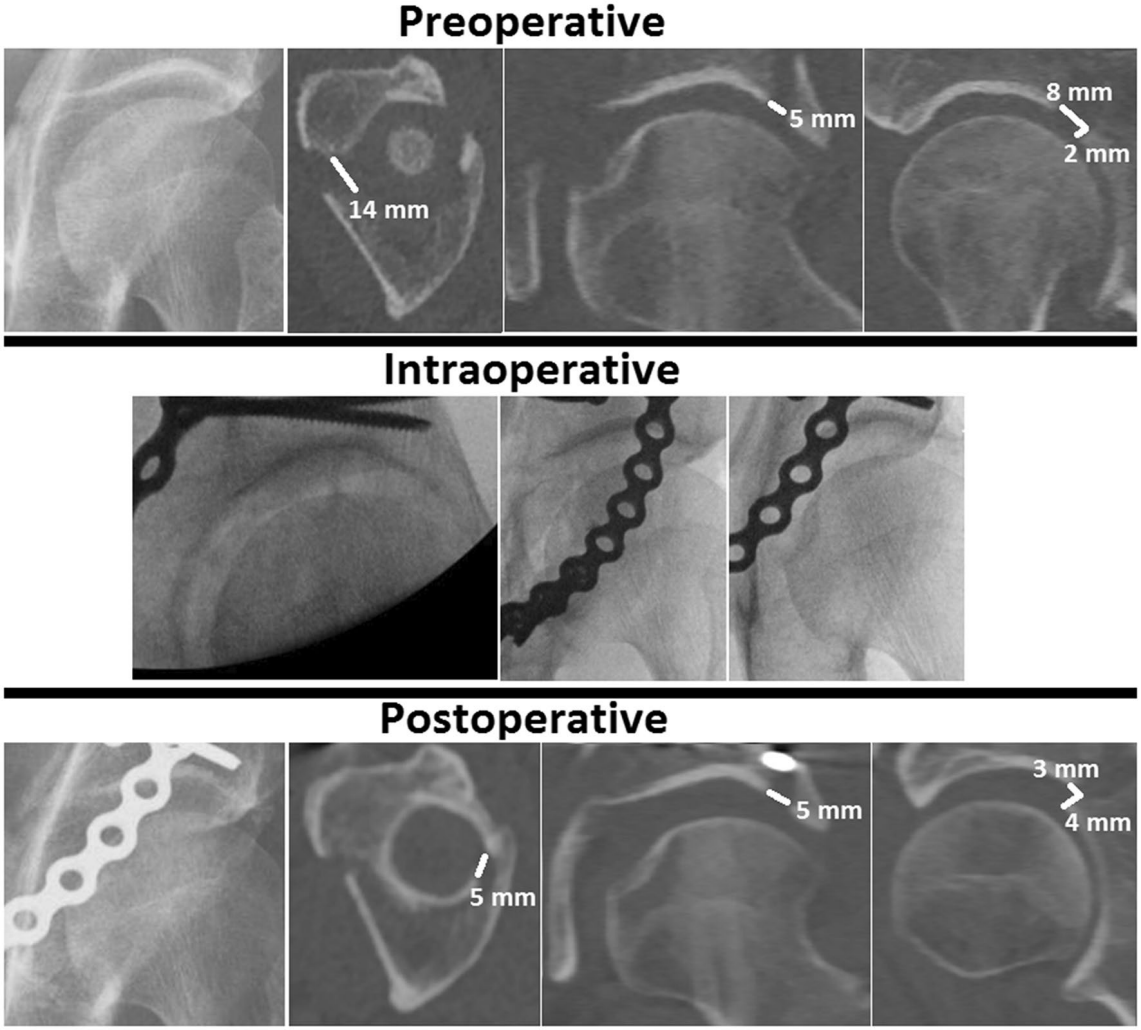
Case example of a both column (AO/OTA 62 C) fracture showing the discrepancy between radiograph, CT scan and intraoperative fluoroscopy images. No gap or step-off was observed on the radiographs and intraoperative fluoroscopy images, whereas the CT images demonstrated gaps and a step-off in all three planes (in white). The preoperative gap was 14 mm on the axial CT slice, 5 mm on the coronal CT slice and 8 mm on the sagittal CT slice. The preoperative step-off was 2 mm, measured on the sagittal CT slice. Postoperatively, a gap of 5 mm (axial and coronal) and 3 mm (sagittal) was measured and the step-off was 4 mm (sagittal).
